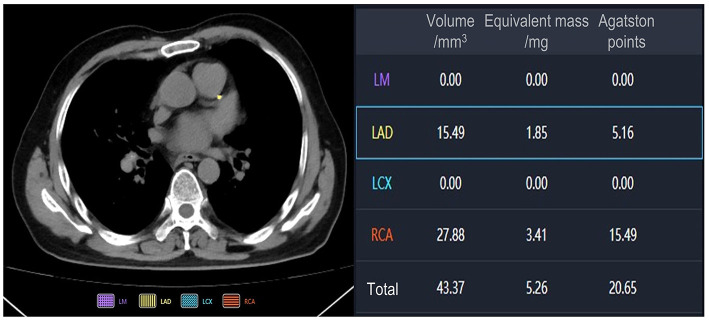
Automated AI quantification of coronary artery calcium (uAI Discover-Nongated Coronary Artery Calcium Score) on a nongated, non-contrast chest CT scan from a 66-year-old male with COPD but without osteoporosis.
